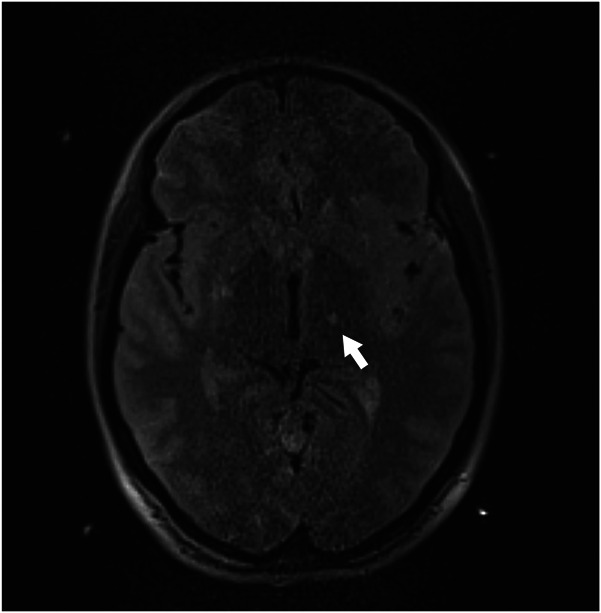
Immediate post‑magnetic resonance‑guided focused ultrasound T2-weighted constructive interference in steady state MRI showing a successful thalamotomy lesion. A typical hyperintense lesion is visible in the ventral intermediate nucleus of the thalamus (white arrow, on the patient's left), confirming acute tissue necrosis and immediate radiographic confirmation of the unilateral ablation procedure.
